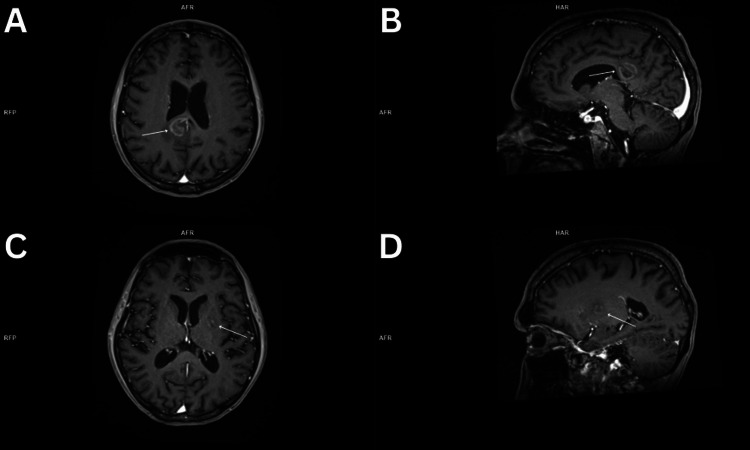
A-D. Brain MRI showing two lesions. (A,B) The larger mass is an expanding heterogeneous lesion in the right frontal lobe measuring 22x23x19 mm surrounded by a small zone of edema.
